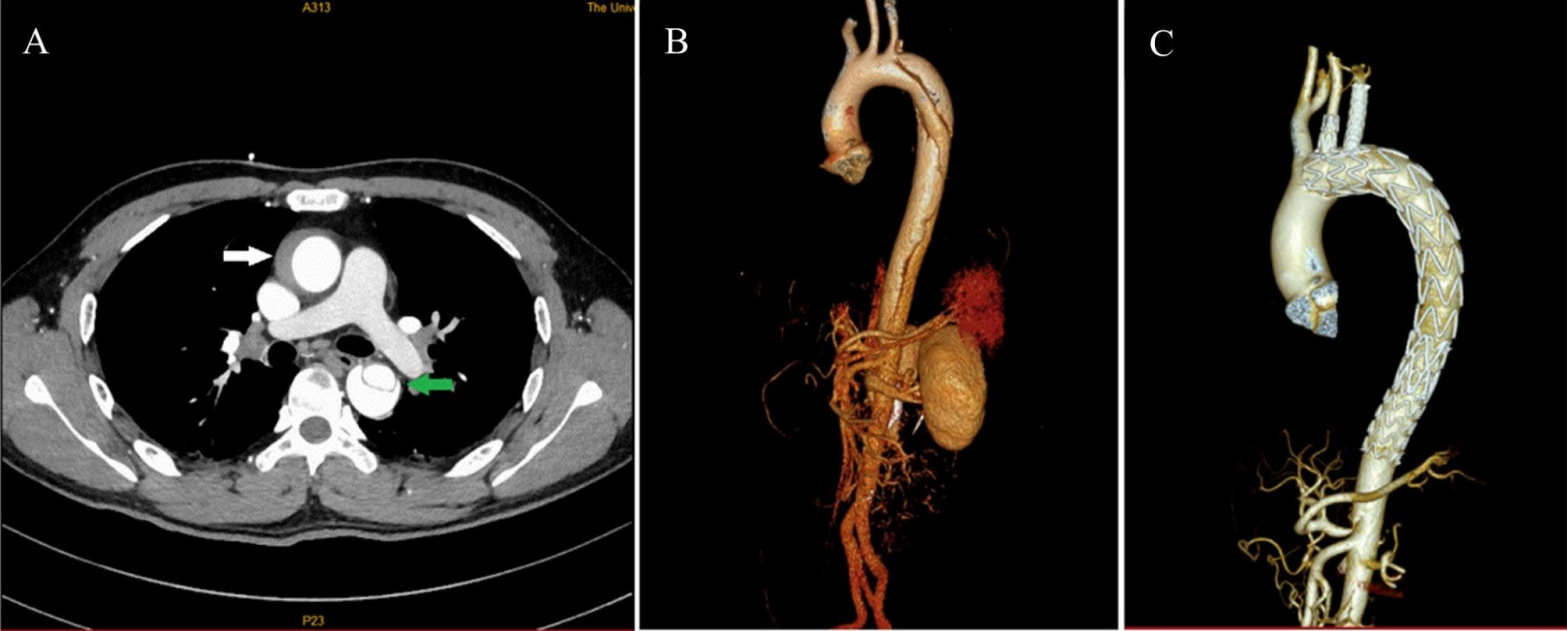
A Preoperative CT scan showing the ascending aortic intramural hematoma (white arrow indicating abnormal soft tissue in the wall of the aorta) and acute type B aortic dissection involving the descending thoracic aorta(green arrow indicating intimal in descending aorta). B Preoperative three-dimensional aortic IMH. C Postoperative three-dimensional imaging of the aorta
